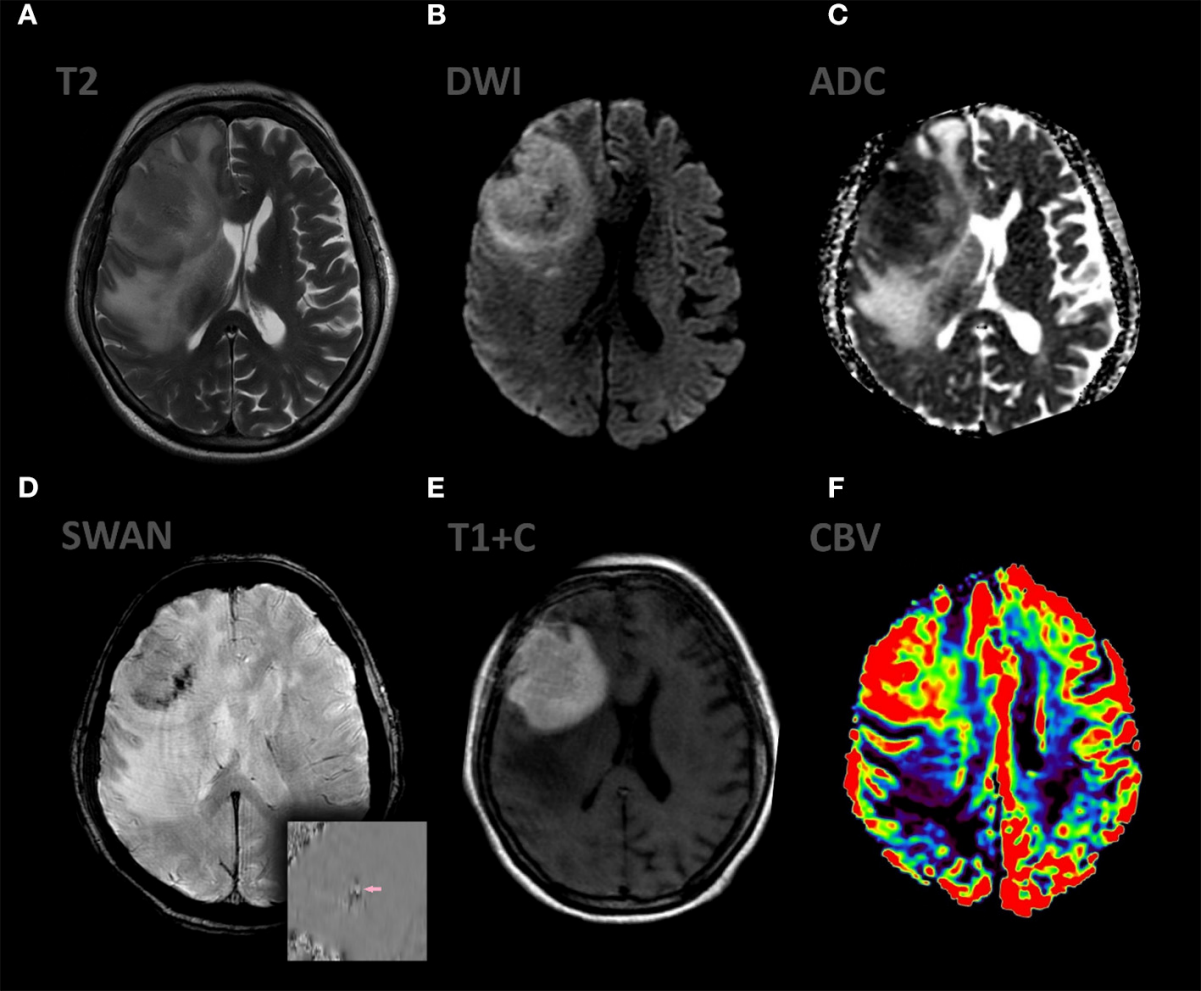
Patient A., female 67 y.o. presented with left sided weakness and altered mental status. MRI ax: (A) T2WI; 6, (B) DWI, (C) ADC, (D) SWAN, (E) T1 + C, (F) DSC-PWI. Axial Brain MRI: there is a large intraaxial mass in right frontal lobe with extensive peritumoral edema on T2WI and mild mass effect. The mass has restricted diffusion and bright, homogeneous contrast enhancement. Most of the tumor exhibits hyperperfusion. SWAN indicates small amounts of hemorrhage.
